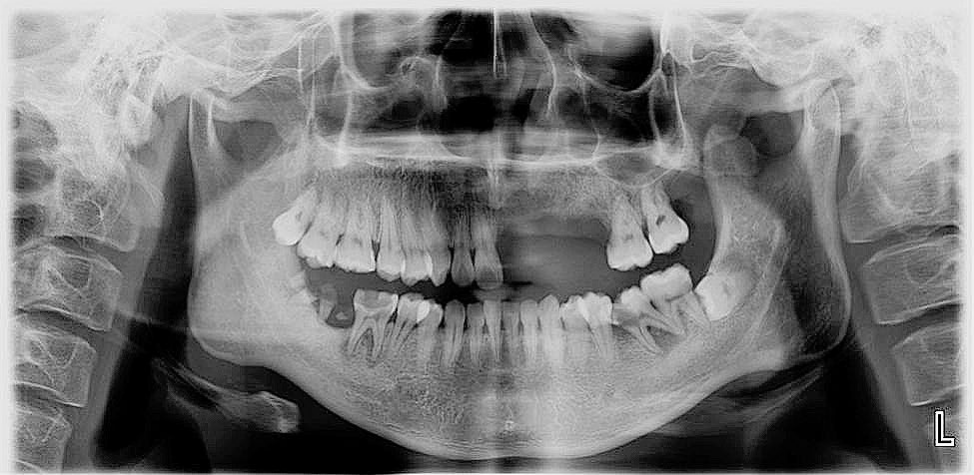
Panoramic radiographs showed the first and second upper left molars in position, accompanied by the loss of distal alveolar bone of the second upper left molar
